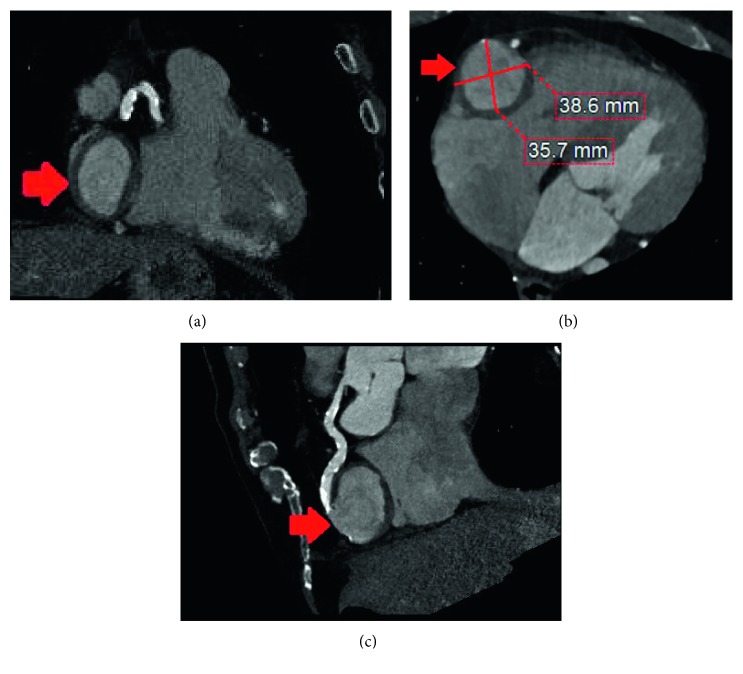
CT coronary angiogram. Thrombosed RCA aneurysm in coronal (a) and axial (b) view in right AV groove. (c) Curved multiplanar reformatted image displaying full length of the RCA. AV: atrioventricular; RCA: right coronary artery.
